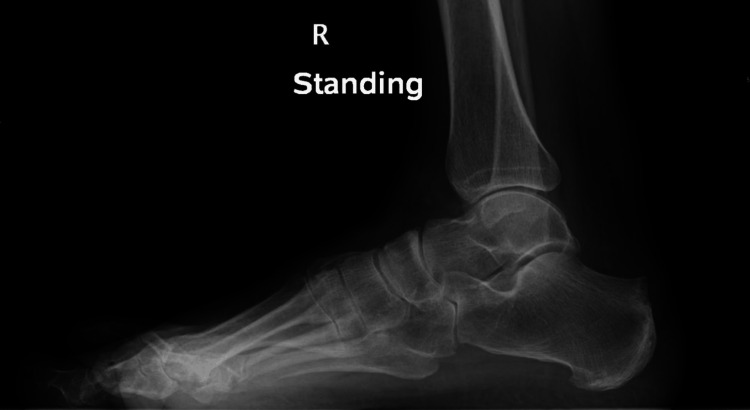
Preoperative radiograph of a symptomatic patient with Haglund’s deformity
